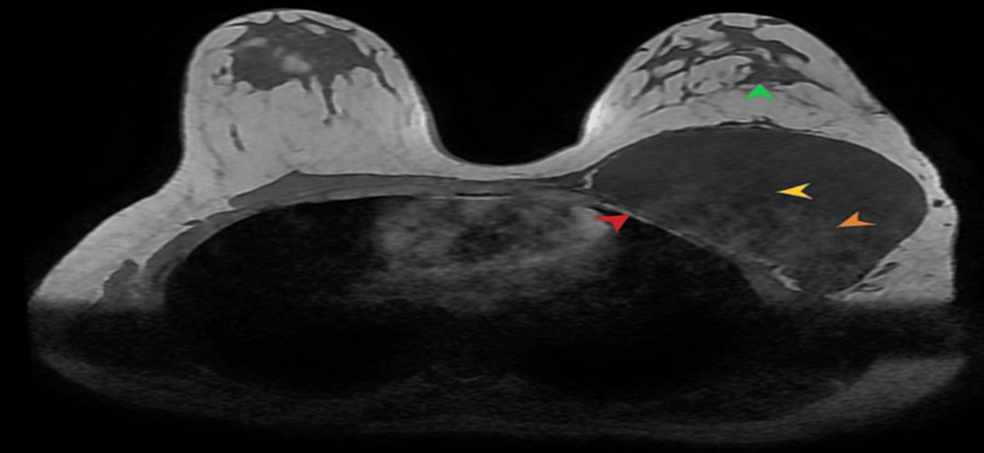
Non-contrast T1 axial MR image shows the lesion attached to left chest wall (annotated by red arrow head). Intralesional T1 low signal areas correspond to necrosis (yellow arrow head), while T1 hyperintense component correlates with hemorrhage (orange arrow head). The left breast parenchyma is displaced anteriorly (green arrow head).
